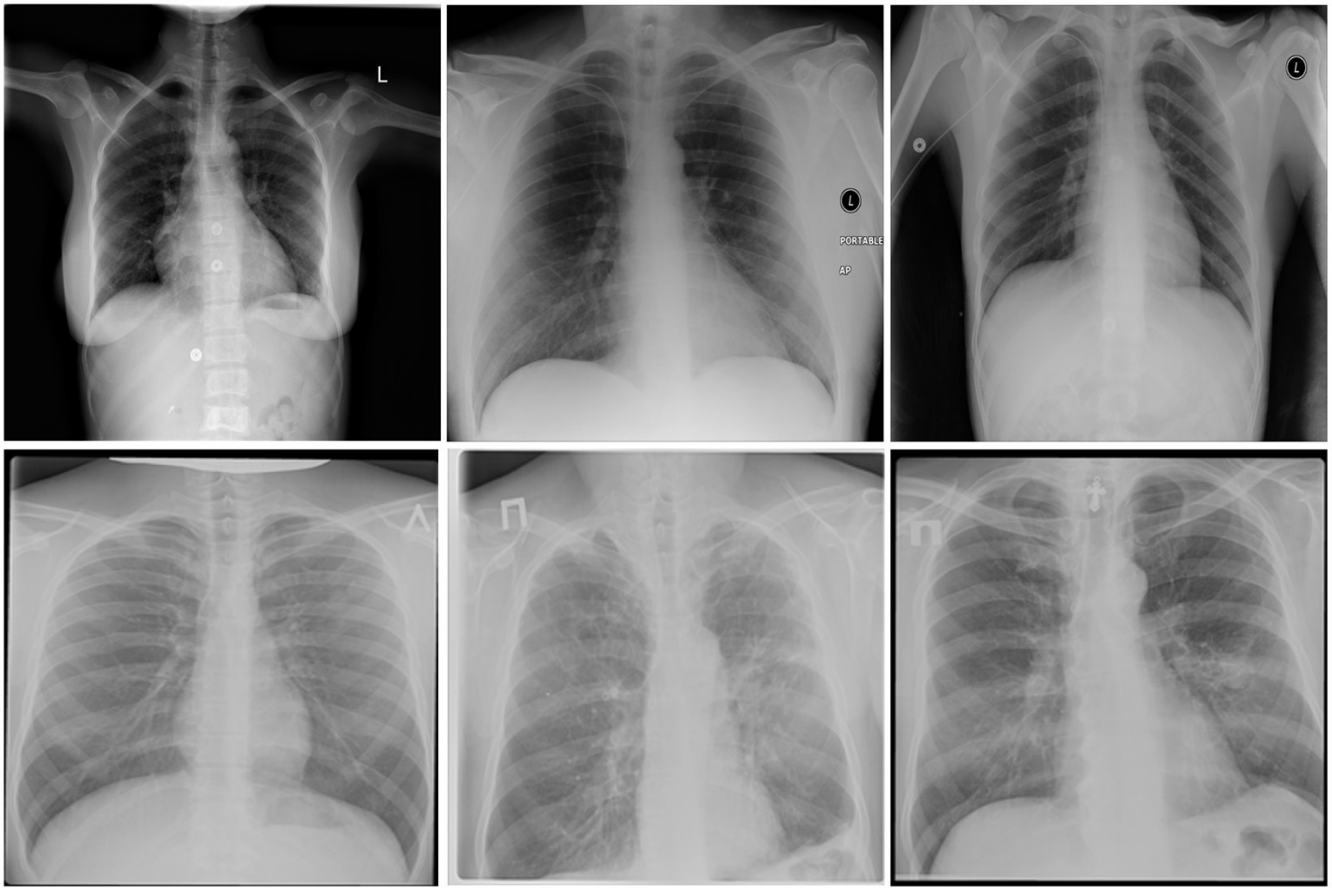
Example chest X-ray images from the multi-national patient cohort introduced by Rahman et al. (2020): (top) TB negative patient cases and (bottom) TB positive patient cases.
